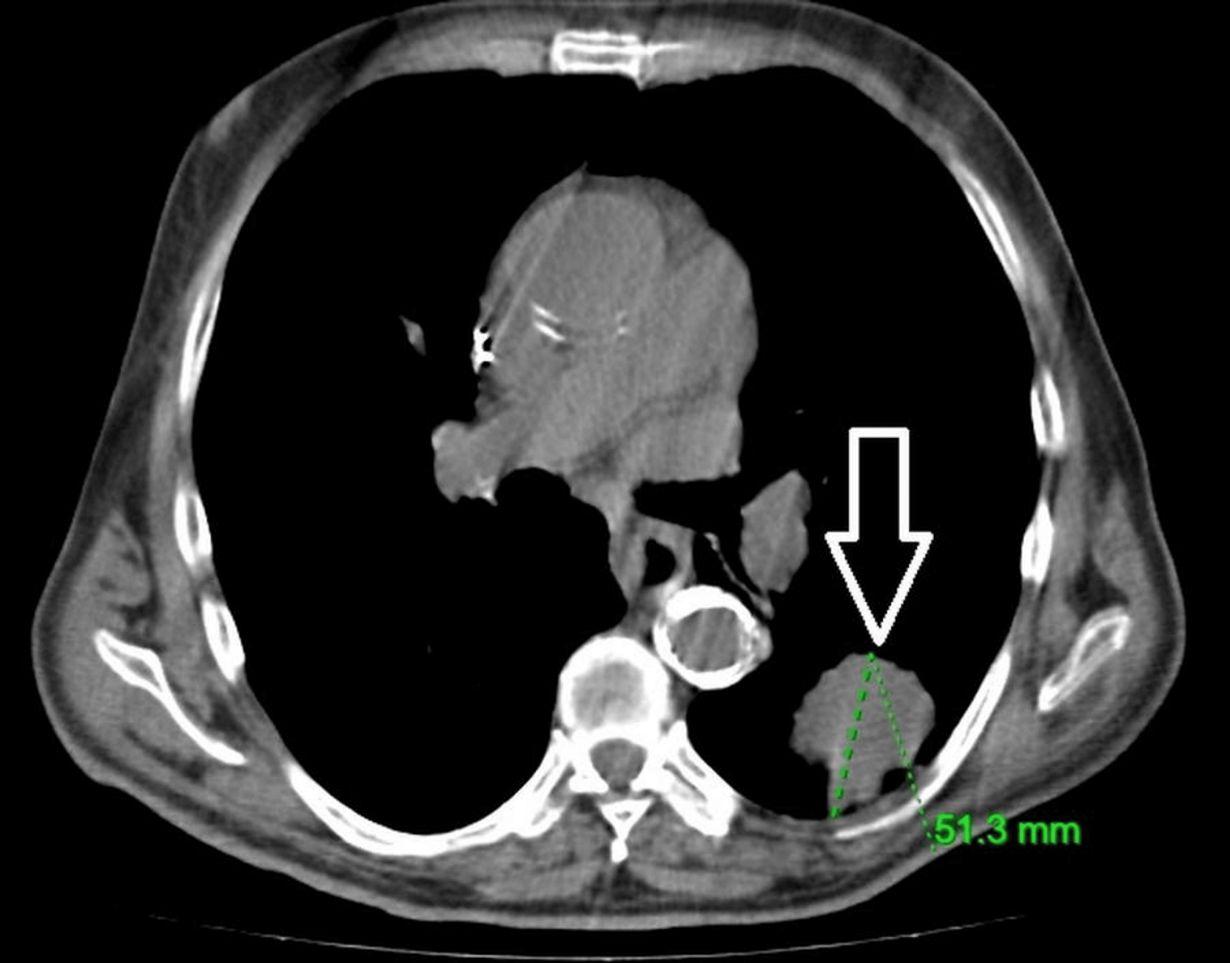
Baseline CT scan CT scan depicting the baseline target lesion measuring 5.1 cm.
